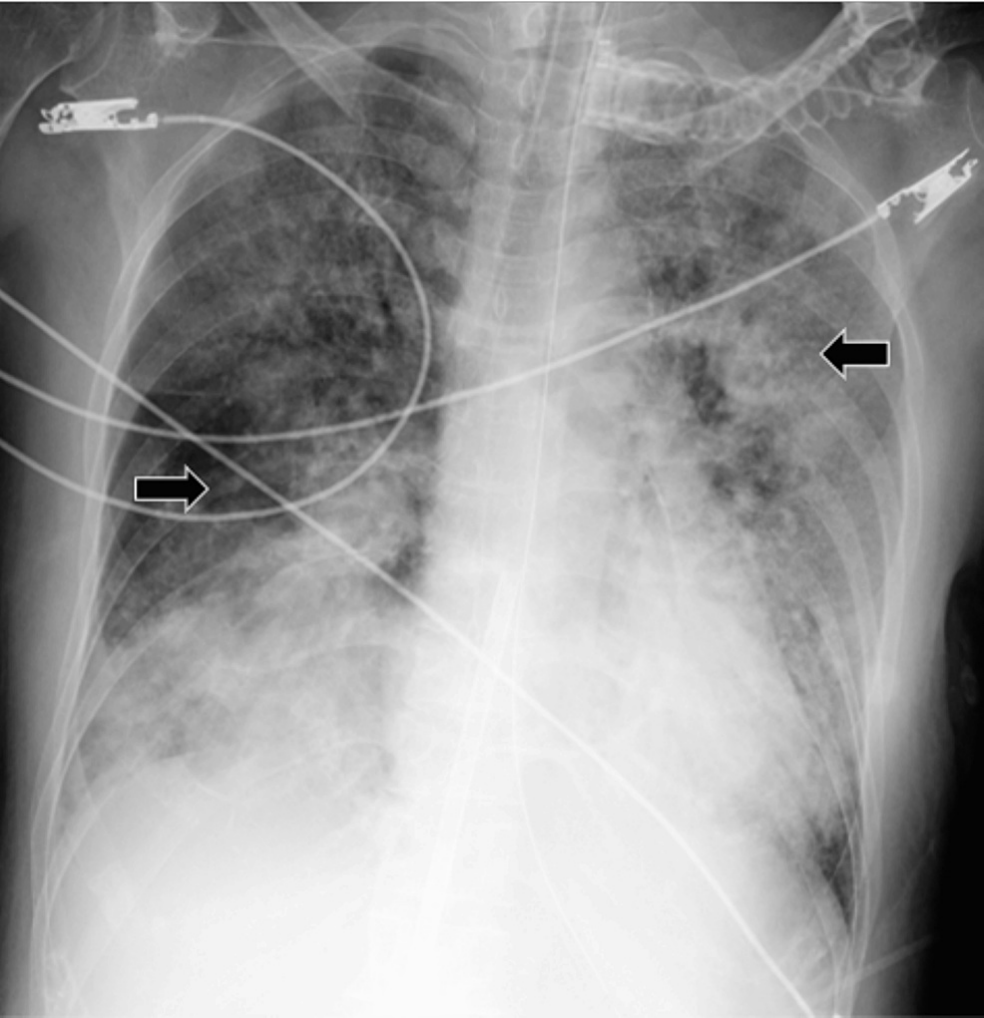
Chest radiography findings on the seventh day Black arrow: chest radiograph reveals severe pulmonary congestion
